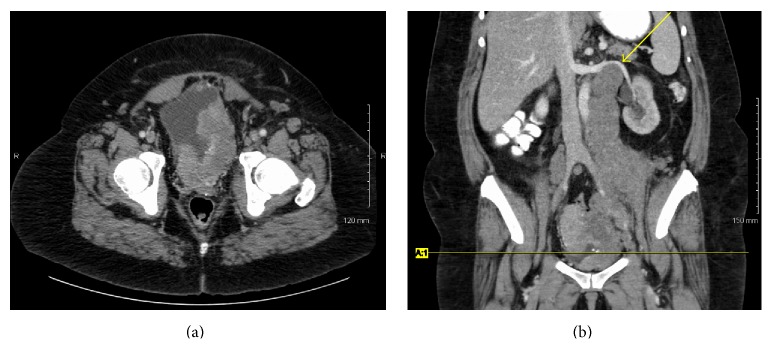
Axial (a) and coronal (b) pelvic CT scan images showing bladder wall thickening and retroperitoneal masses displacing left renal vein (arrow). The axial image in (a) is at the level of the horizontal line noted in (b).
